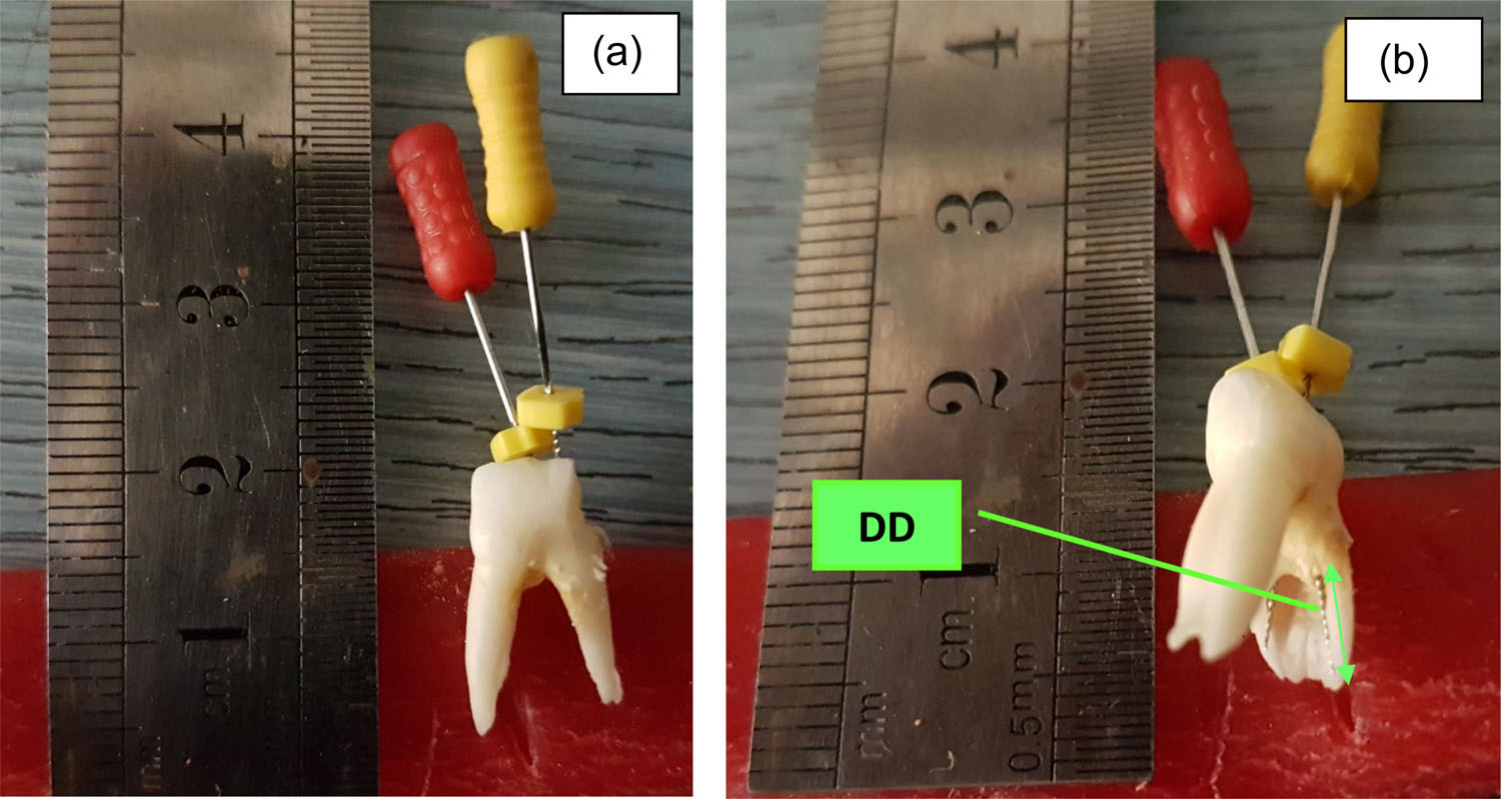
Difference of root resorption degree between the out and inside aspects of the primary root*. Inserting K‐files in the distobuccal and distolingual canal of the first lower primary molar with moderate resorption (as it appears in the radiograph) (a), the difference distance (DD) is more than 4 mm in the same root because the resorption degree is more than half of the root in the inside aspect (b).
